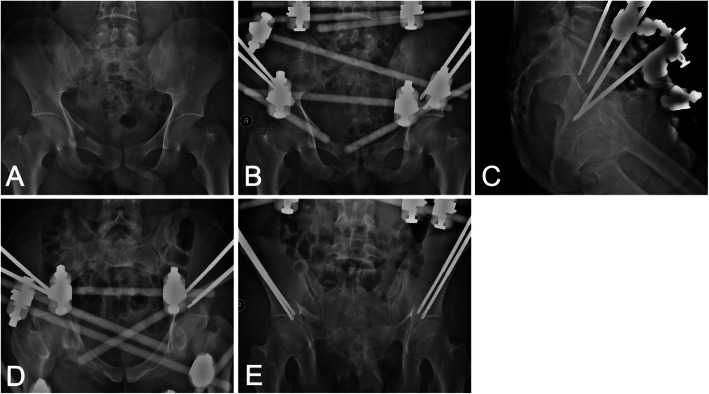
Preoperative and postoperative X-ray of the pelvis. a Preoperative X-ray, b Postoperative X-ray in the AP plane, c Postoperative X-ray in the lateral projection, d Postoperative X-ray in the inlet projection, e Postoperative X-ray in the outlet projection
